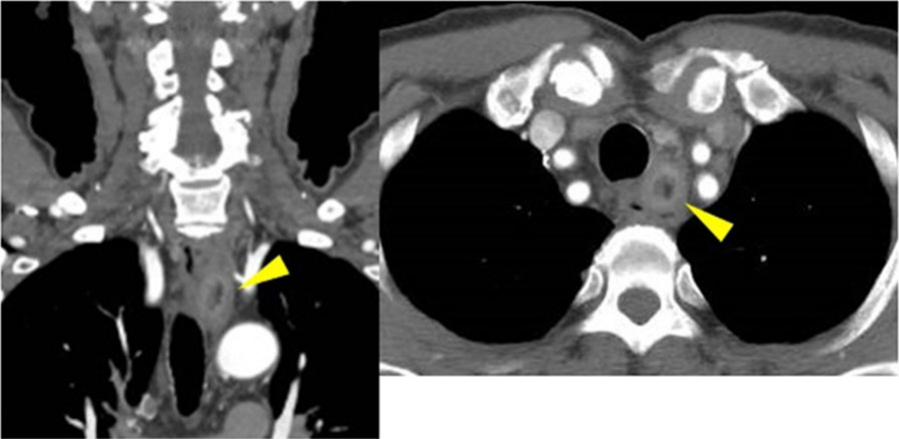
Contrast-enhanced CT. A superior mediastinal foreign body, located between trachea and esophagus, was sticking toward the internal, caudal side (arrowhead)
